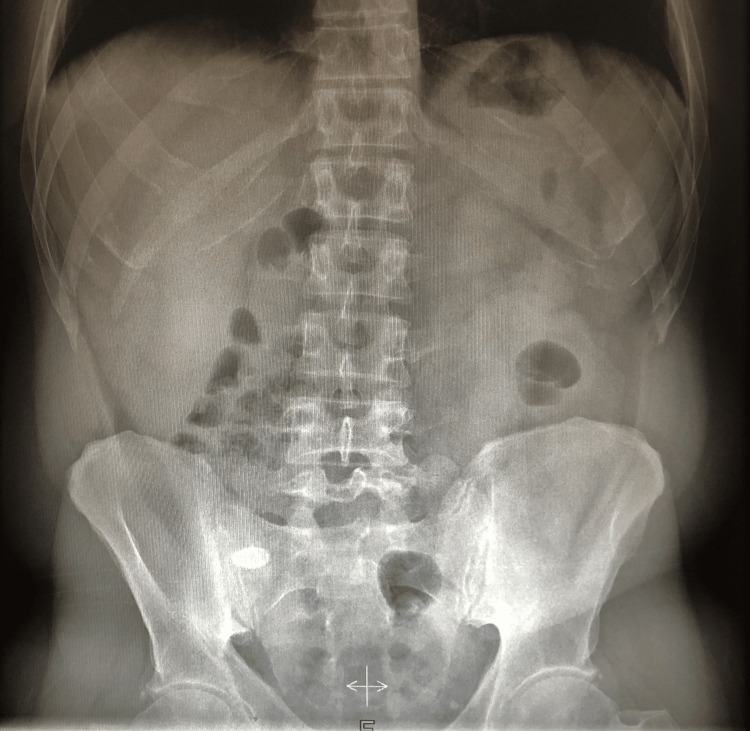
Abdominal X-ray showed a radiopaque finding.
